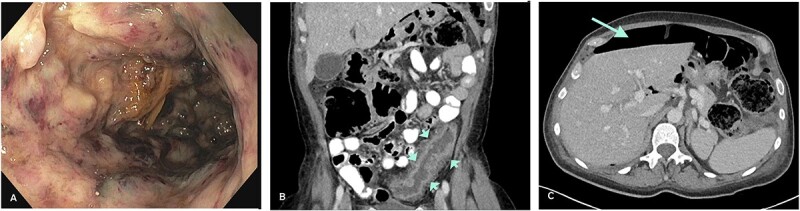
(A) Severe colitis with deep ulcerations on endoscopy. (B) Left-sided colonic wall thickening (CT-scan; short arrows). (C) Intraperitoneal air indicative of hollow viscus perforation (CT-scan; long arrow).
